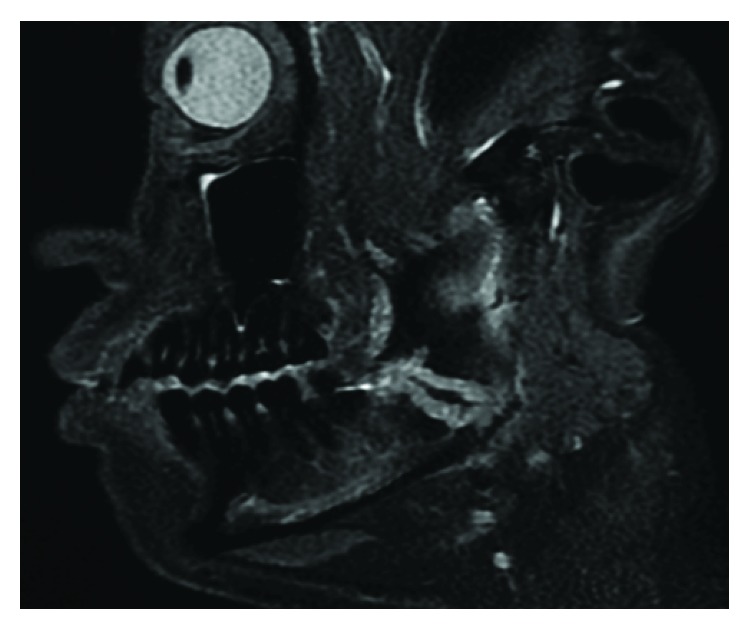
Sagittal magnetic resonance imaging (MRI) obtained during the initial visit. MRI shows that the mandibular bone marrow from the right ramus to the body has a high signal density on short-tau inversion recovery (STIR) images and the cortical bone is partially raptured. Moreover, filaments in the low-density area are observed in the high-density area.
